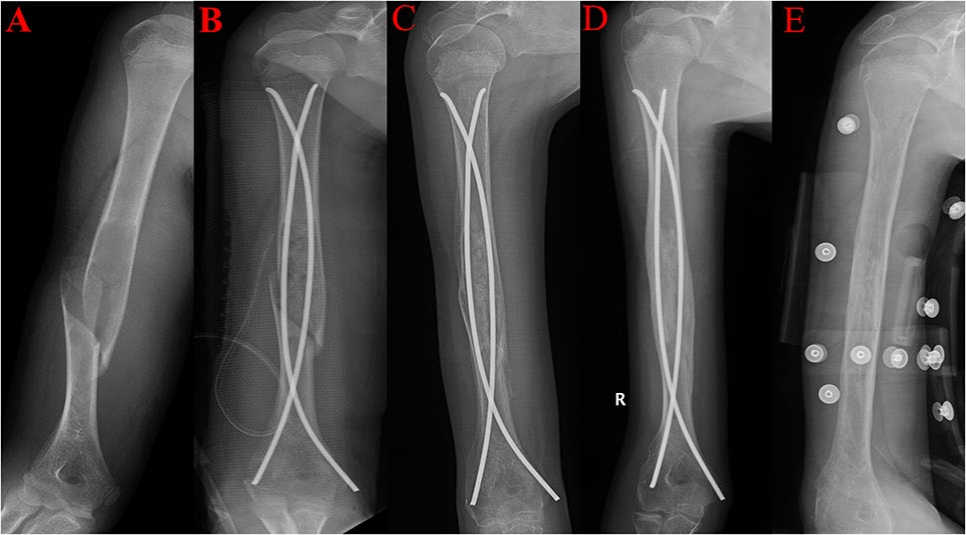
A 12-year-old male with pathological fracture of the right humerus. (A) The fracture before surgery on radiograph. (B) Right humerus lesion removal, bone grafting, and two elastic stable intramedullary nails fixation. (C) The fracture started to heal 2 months postoperatively. (D) The simple bone cyst and fracture was healed 7 months postoperatively. (E) Two nails were removed successfully, and the surgery duration was 10 min.
